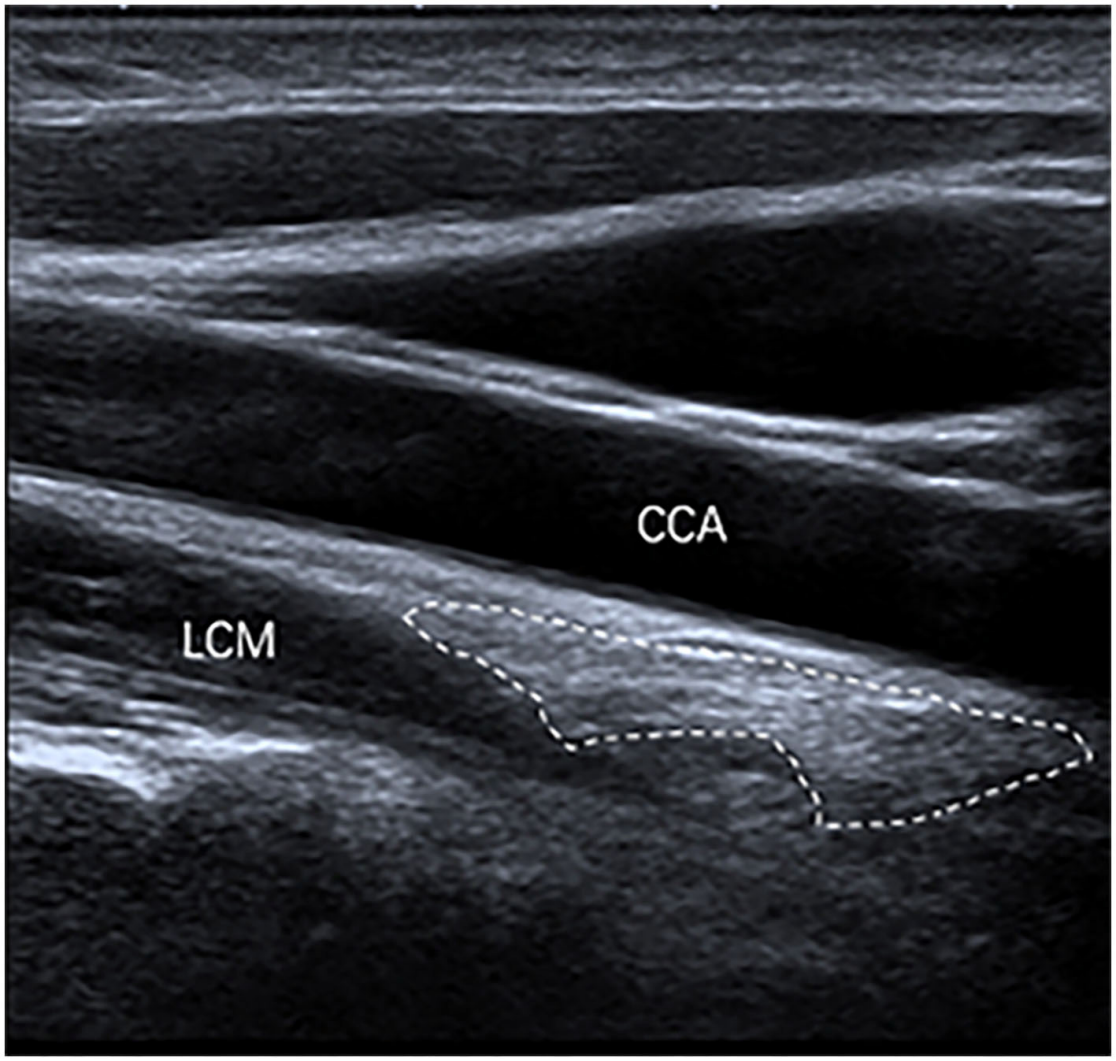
The SG, with a dumbbell shape (outlined) and hyperechogenicity, was located between the common carotid artery (CCA) and the longus colli muscle (LCM) on the longitudinal ultrasound image.
